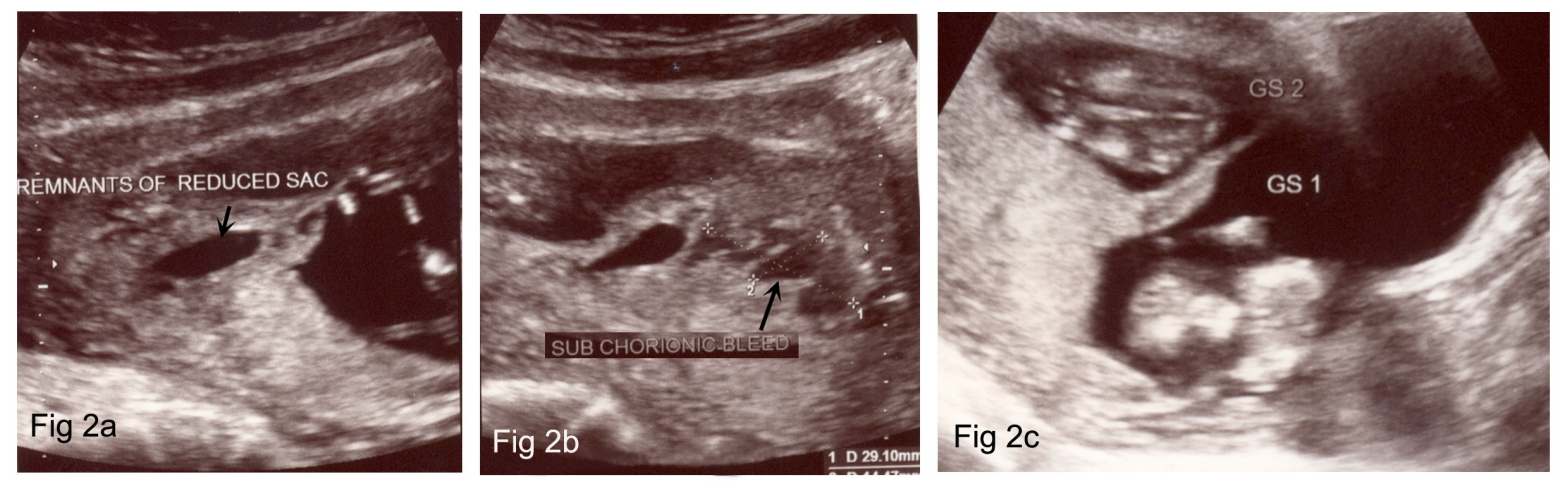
An ultrasound image showing remnants of reduced fetal sac (Fig 2a), sub-chorionic bleed (Fig 2b) and two fetal sacs resulting in twin delivery (Fig 2c)
